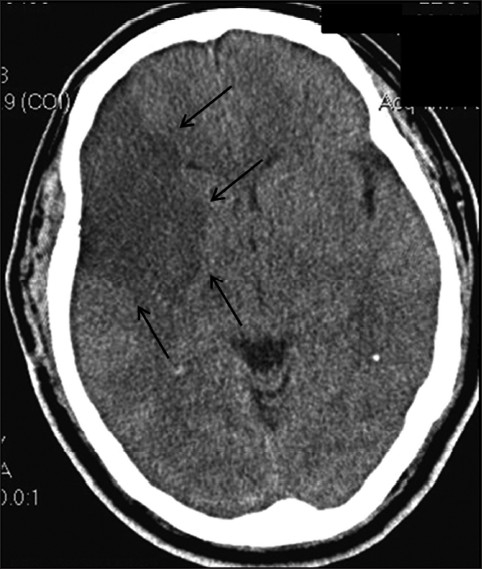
Axial CT scan of the brain without contrast 20 hours after completing prostatectomy demonstrates right MCA territory infarction with 3-mm midline shift
